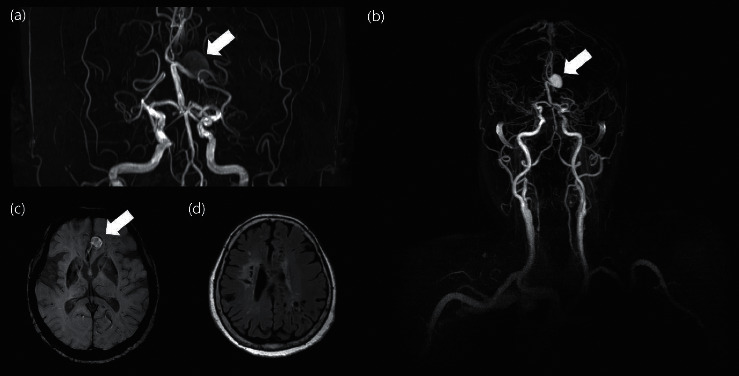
The brain MR images in moyamoya disease patients with a large intracranial aneurysm. (a) Brain TOF-MRA presenting a bilateral middle cerebral artery occlusion with a large aneurysm (arrow). (b) Brain MRA with gadolinium enhancement in the same patient with a large aneurysm (arrow). (c) SWI image presenting with large intracranial aneurysms (arrow). (d) FLAIR image showing multiple old ischemic lesions in the parenchyma.
